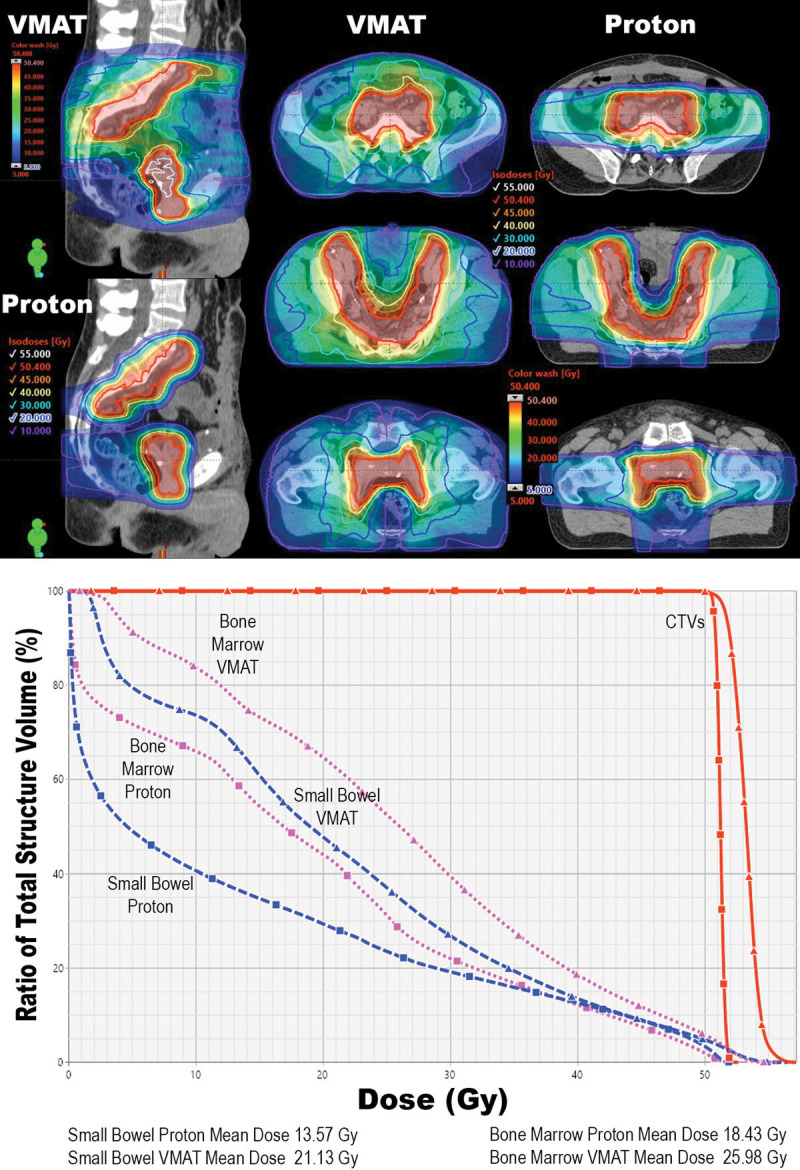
Sagittal and axial computed tomography slices of a study patient including radiation isodose lines and dose color wash of the delivered VMAT plan and a comparative PBT plan (top) and the representative DVH graphs (bottom). This patient with high-risk bladder cancer received postoperative radiation therapy to 50.4 Gy. Abbreviations: VMAT, volumetric moderated arc therapy; PBT, proton beam therapy; DVH, dose-volume histogram.
